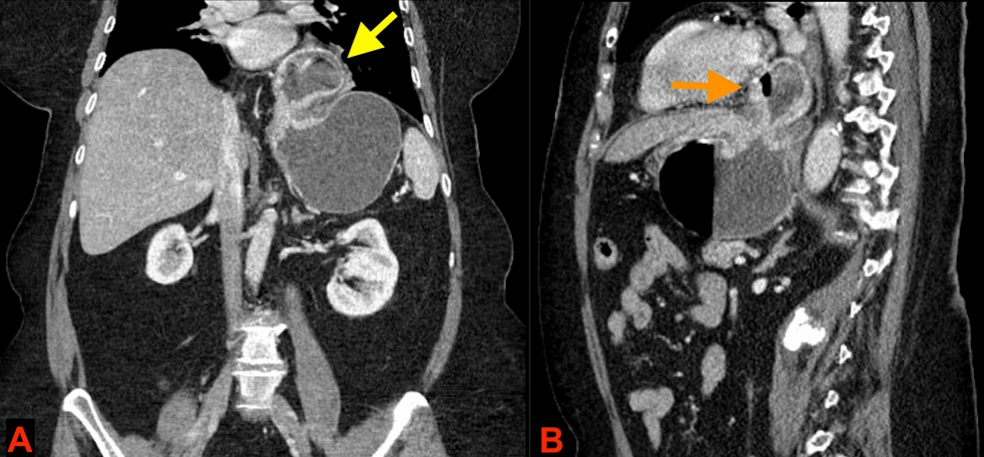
CT abdomen and pelvis with IV contrast demonstrating a large hiatal hernia with a suspected mesenteroaxial gastric volvulus. A: Coronal view, and B: sagittal view. Yellow arrow in image A shows gastric body in the hiatus. Orange arrow in image B shows gastric body located in the posterior mediastinum.
